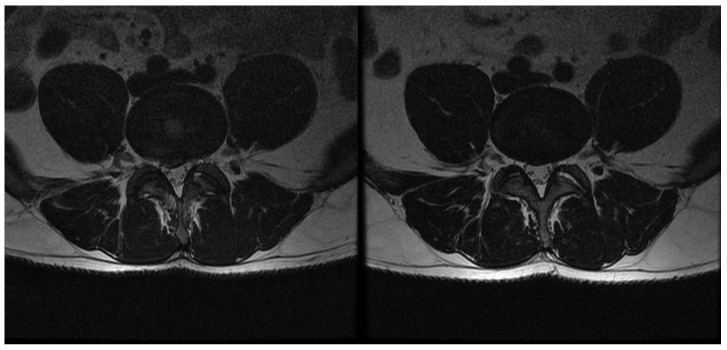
Pre- (left) and post-GEI (right) axial MRI at L4/L5 level of of patient with radiculopathy. MRI was performed four weeks before and six weeks after the GEI.
